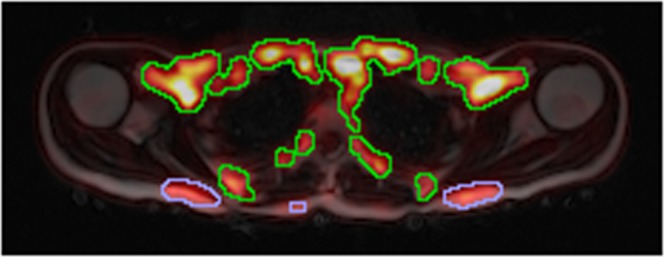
Axial slice showing the bilaterally symmetric FDG uptake in the posterior subcutaneous adipose tissue. MRI-derived fat signal image from the dual-echo scan in grayscale with the PET-derived GUR superimposed using the heat colourmap. The blue lines delineate the posterior subcutaneous adipose tissue areas with a GUR > 10 μmol/100 ml/min. The green lines delineate the remaining BAT areas with a GUR > 10 μmol/100 ml/min.
